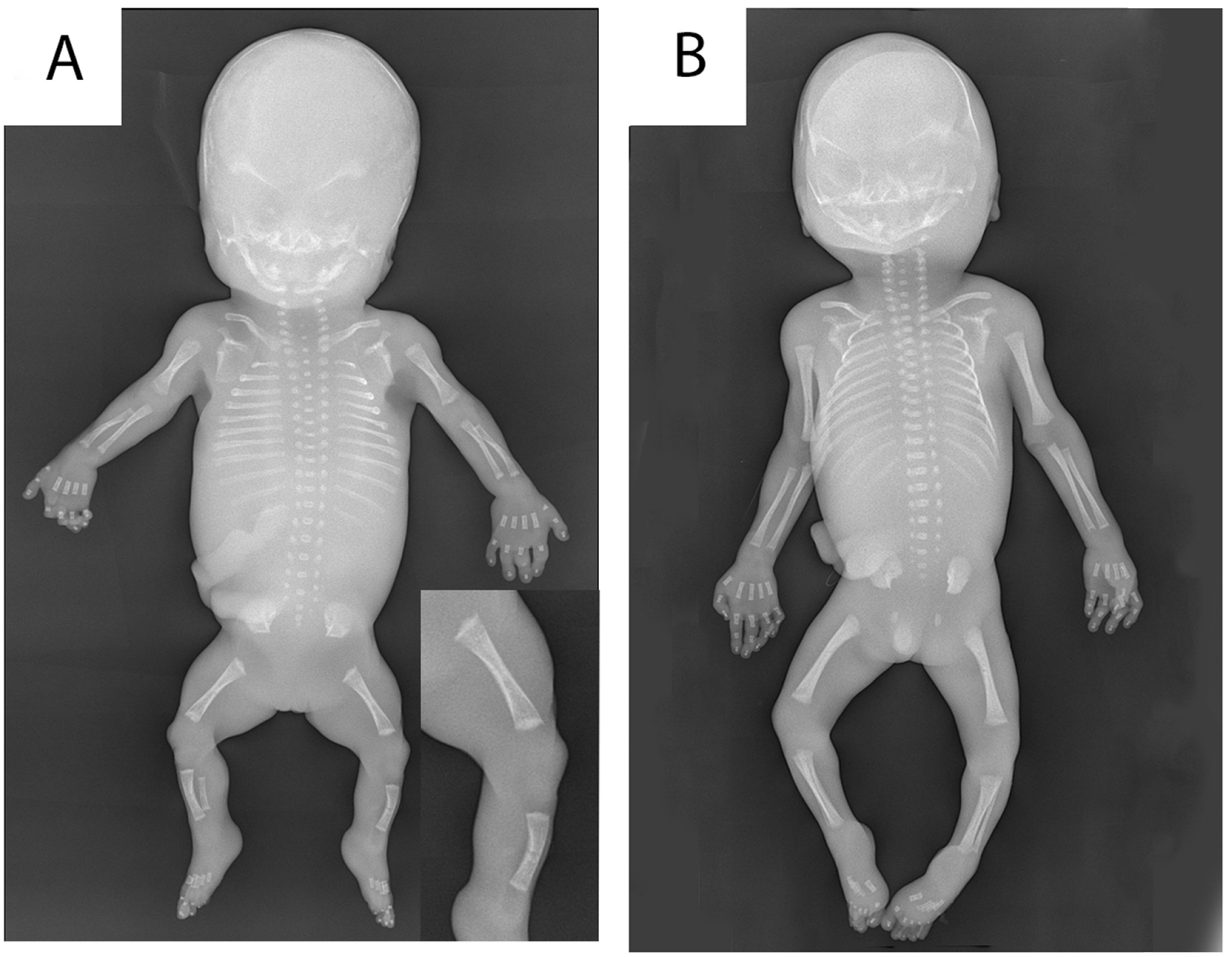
Radiographic examination of our patient with *ARCN1*-related syndrome (A) compared with an age-matched control (B) showed short lengths of long bones, irregular epiphyses (inset), short ribs, platyspondyly, and slightly reduced ossification of the diaphyses, skull, vertebral bodies, and pubic bones.
